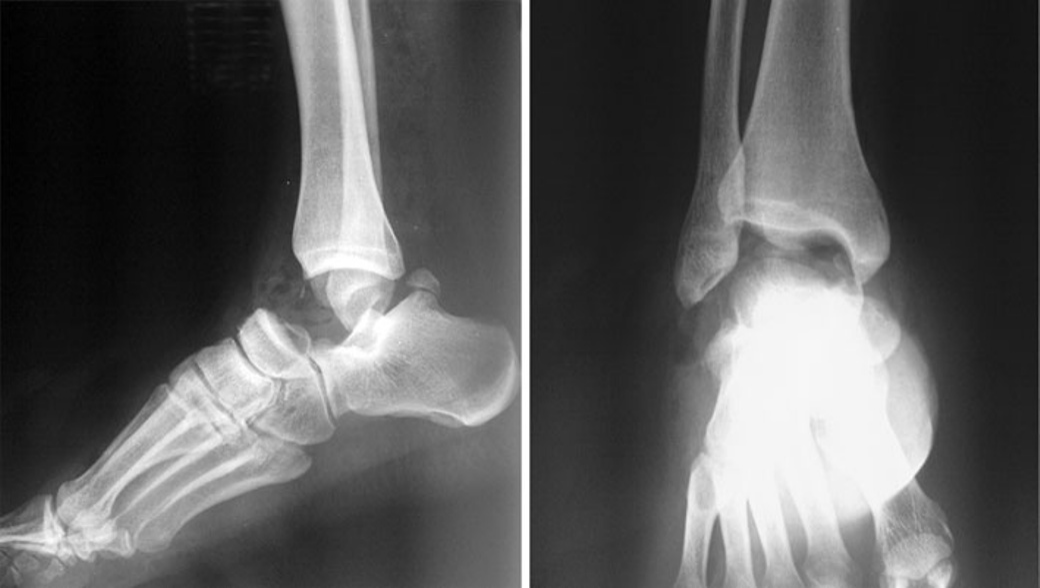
X-ray shows the absence of talus without any fracture of the surrounding bones
